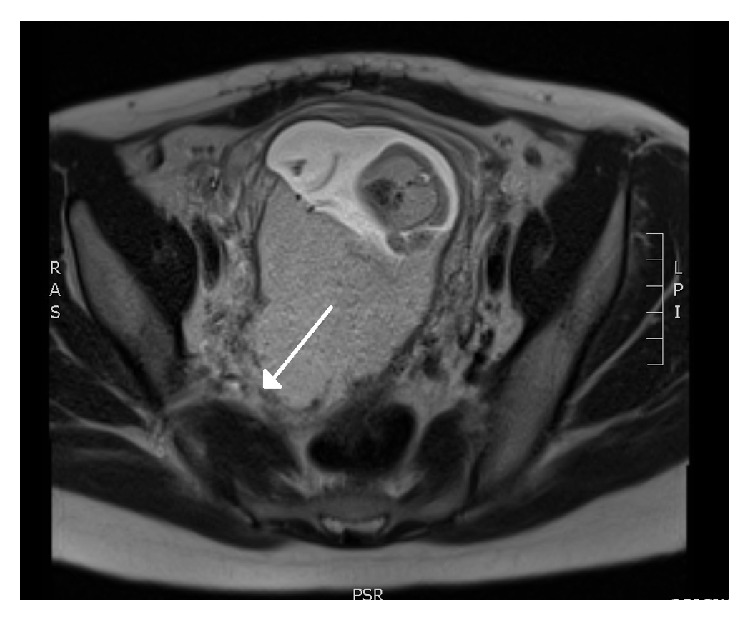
MRI demonstrating placental invasion into the myometrium (arrow).
